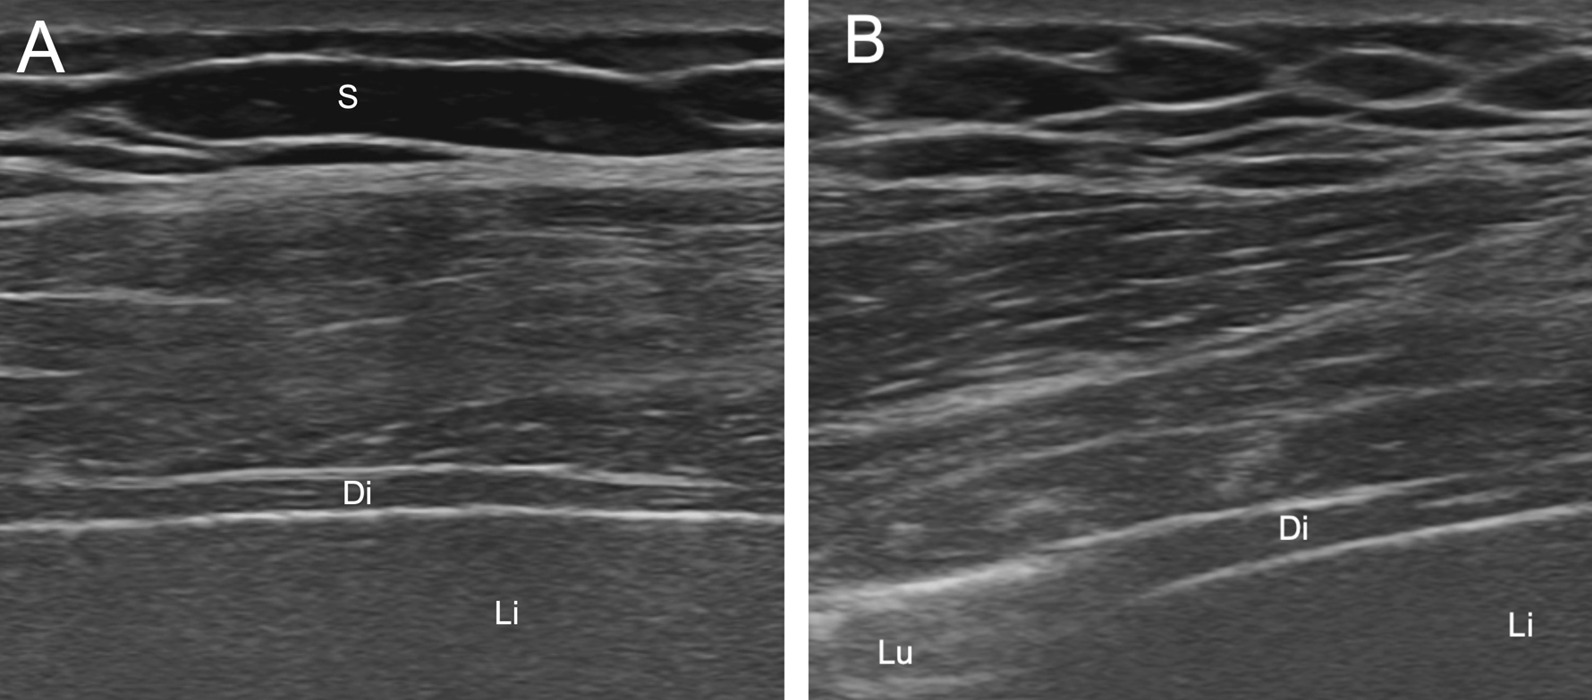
B-mode ultrasound of the right hemidiaphragm. A ultrasound performed during end-expiration. B Ultrasound performed during maximal inspiration. The diaphragm is visible between two-hyperechoic lines representing the pleural and peritoneal membranes, respectively. At the end of maximal inspiration, lung tissue is visible in B, end-expiration diaphragm thickness was 1.90 mm. *Di* diaphragm, *S* subcutaneous tissue, *Li* liver, *Lu* lung
